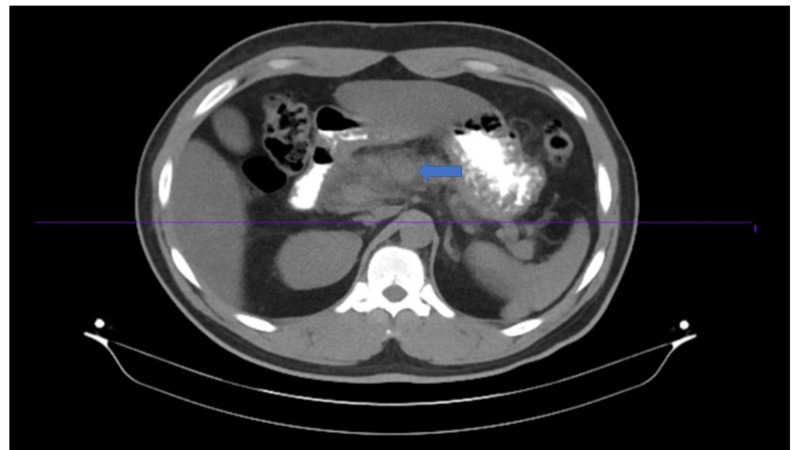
Cross-sectional image of CT abdomen with blue arrow showing the inflammation surrounding the pancreas compatible with acute pancreatitis.
